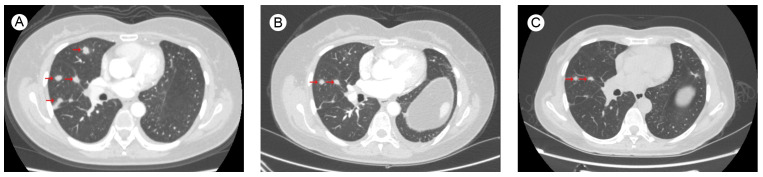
Chest CT images of lung metastases (A) before Almonertinib + Pyrotinib treatment in December 2022; during Almonertinib + Pyrotinib treatment (B) in March 2023; and (C) in November 2023.
